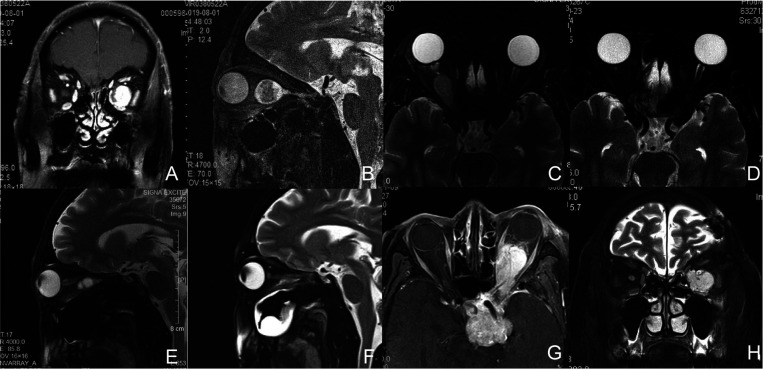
Magnetic resonance (MR) images of Patient1, 2, 3, and 5. (A,B) Preoperative coronal (A) and sagittal (B) T2-weighted images of Patient 1 with a left intraconal schwannoma medial to the optic nerve. (C,D) Axial T2-weighted images of Patient 2 with a right intraconal meningioma involving the intracanalicular segment of the optic nerve preoperatively (C) and 2 months postoperatively (D). (E,F) Sagittal T2-weighted images of Patient 3 with a right intraconal schwannoma preoperatively (E) and 2 months postoperatively (F). (G,H) Axial T1-weighted (G) and coronal T2-weighted (H) images of Patient 5 with a recurrent pituitary tumor involving the sellar region, suprasellar region, cavernous sinus, intraconal space, infratemporal fossa, and pterygopalatine fossa.
